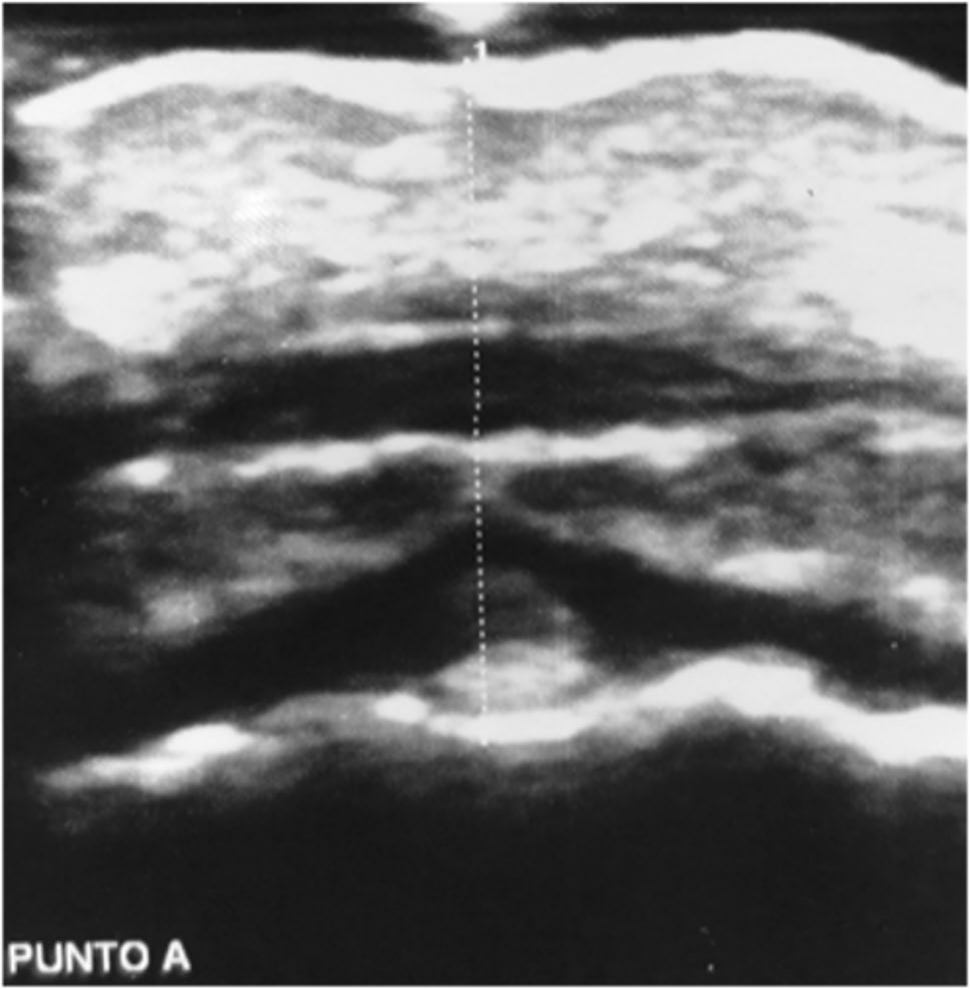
Ultrasonographic soft tissue thickness over A cephalometric point
